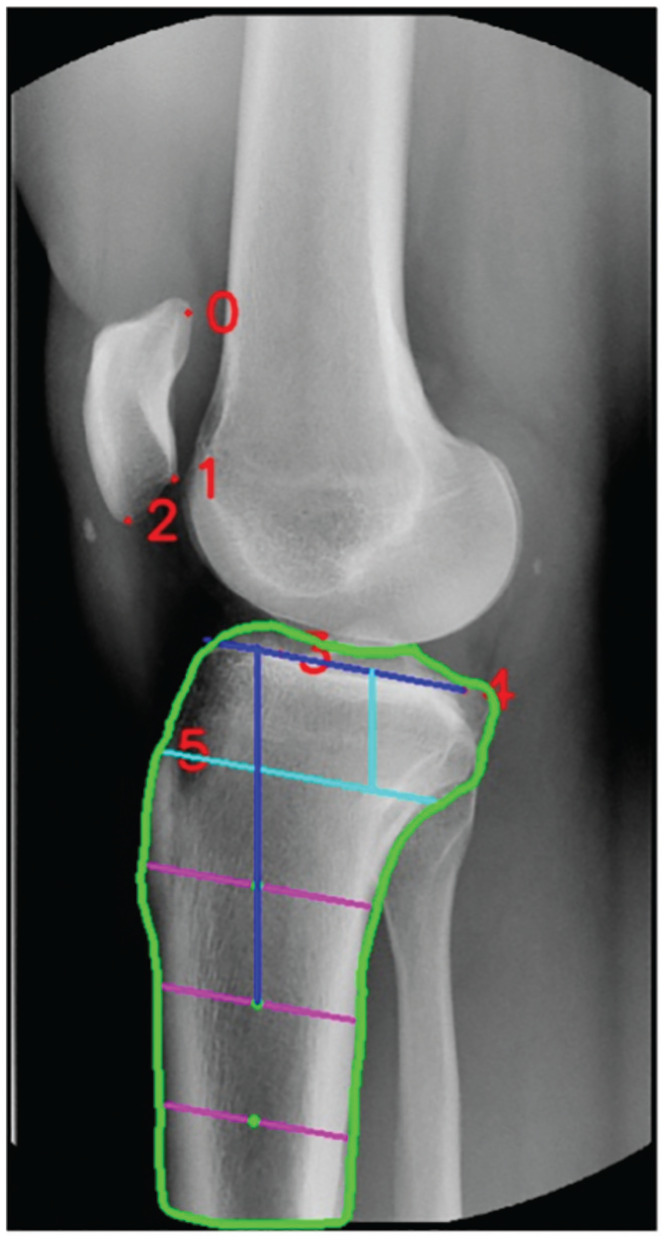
Automated measurement of the posterior tibial slope on a short lateral knee radiograph using the 2A/3A method, demonstrating a slope of 11°. The green outline represents the automated identification of the tibial cortices. The vertical light blue line represents the distance A, defined as the vertical distance from the tibial joint line to the proximal tibial tubercle. The 3 pink lines represent the distances of 2A, 3A, and 4A from the tibial joint line. The midpoints of the pink lines are annotated with a green dot, which is used to determine the tibial shaft axis, in this case using the 2A and 3A lines. The dark blue lines represent the posterior tibial slope, defined as the angle between the tibial shaft axis and the medial tibia joint line. The bony landmarks are labeled as follows: 0, proximal patella; 1, distal patellar articular surface; 2, distal pole of patella; 3, anteriomedial proximal tibia; 4, posteromedial proximal tibia; 5, proximal tibial tubercle.
